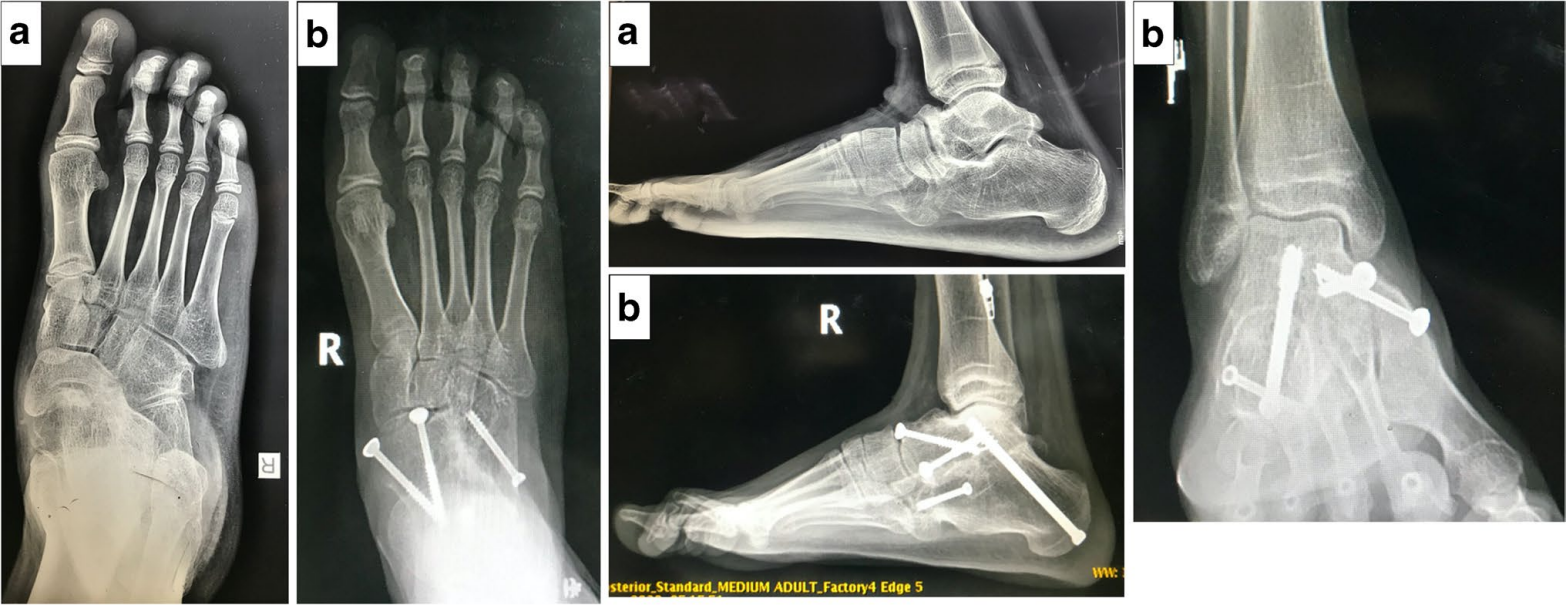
Pre-operative (a) and 12 months post-operative (b) standing foot radiographs of a triple arthrodesis. This 16-year-old male patient with right-sided PTTI stage III had a significant improvement in his AOFAS hindfoot score from 62 pre-operatively to 90 post-operatively. Union was achieved at 2.5 months. No complications were reported, and the surgical time was 120 min
